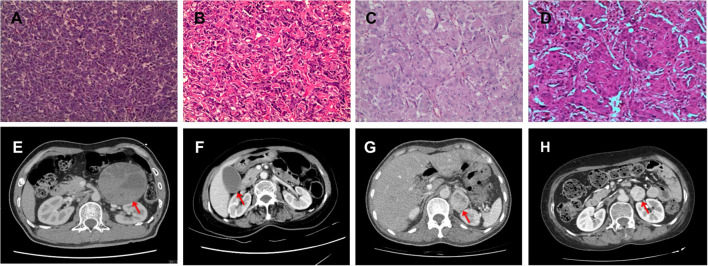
Pathology and CT images of the tumor (A,E): Patient 1; (B,F): Patient 2; (C,G): Patient 3; (D,H): Patient 4. Red arrow indicate tumor mass.
